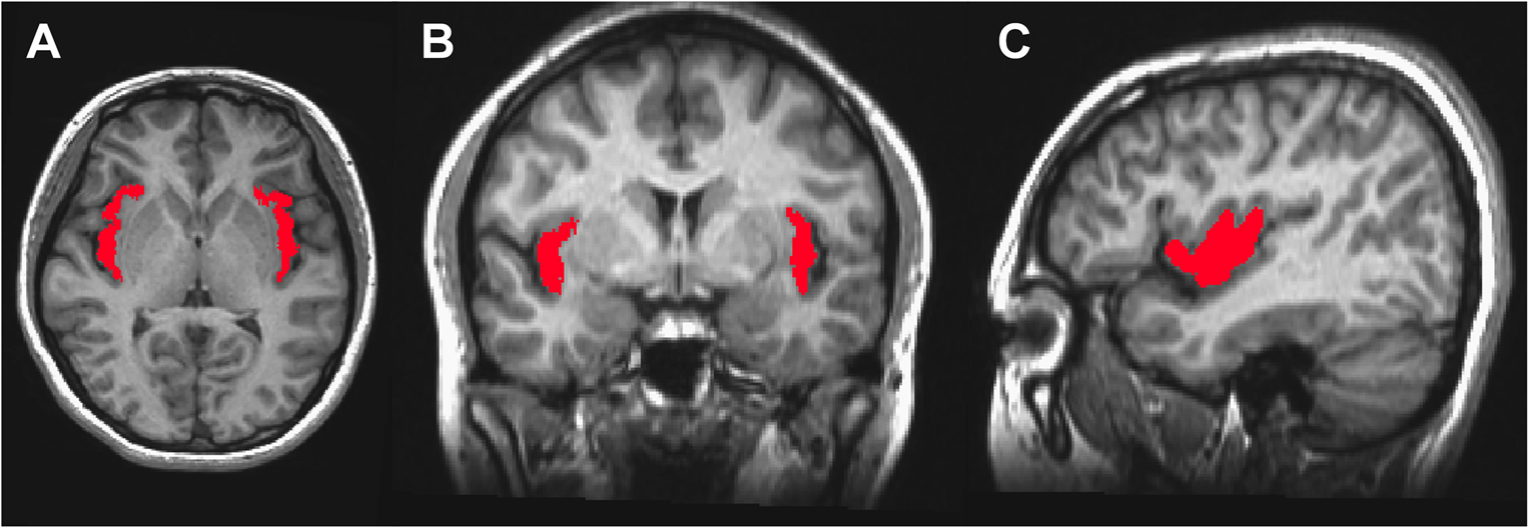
Left and right insular cortex manually defined on T1-weighted MRI in a axial, b coronal, and c sagittal view
